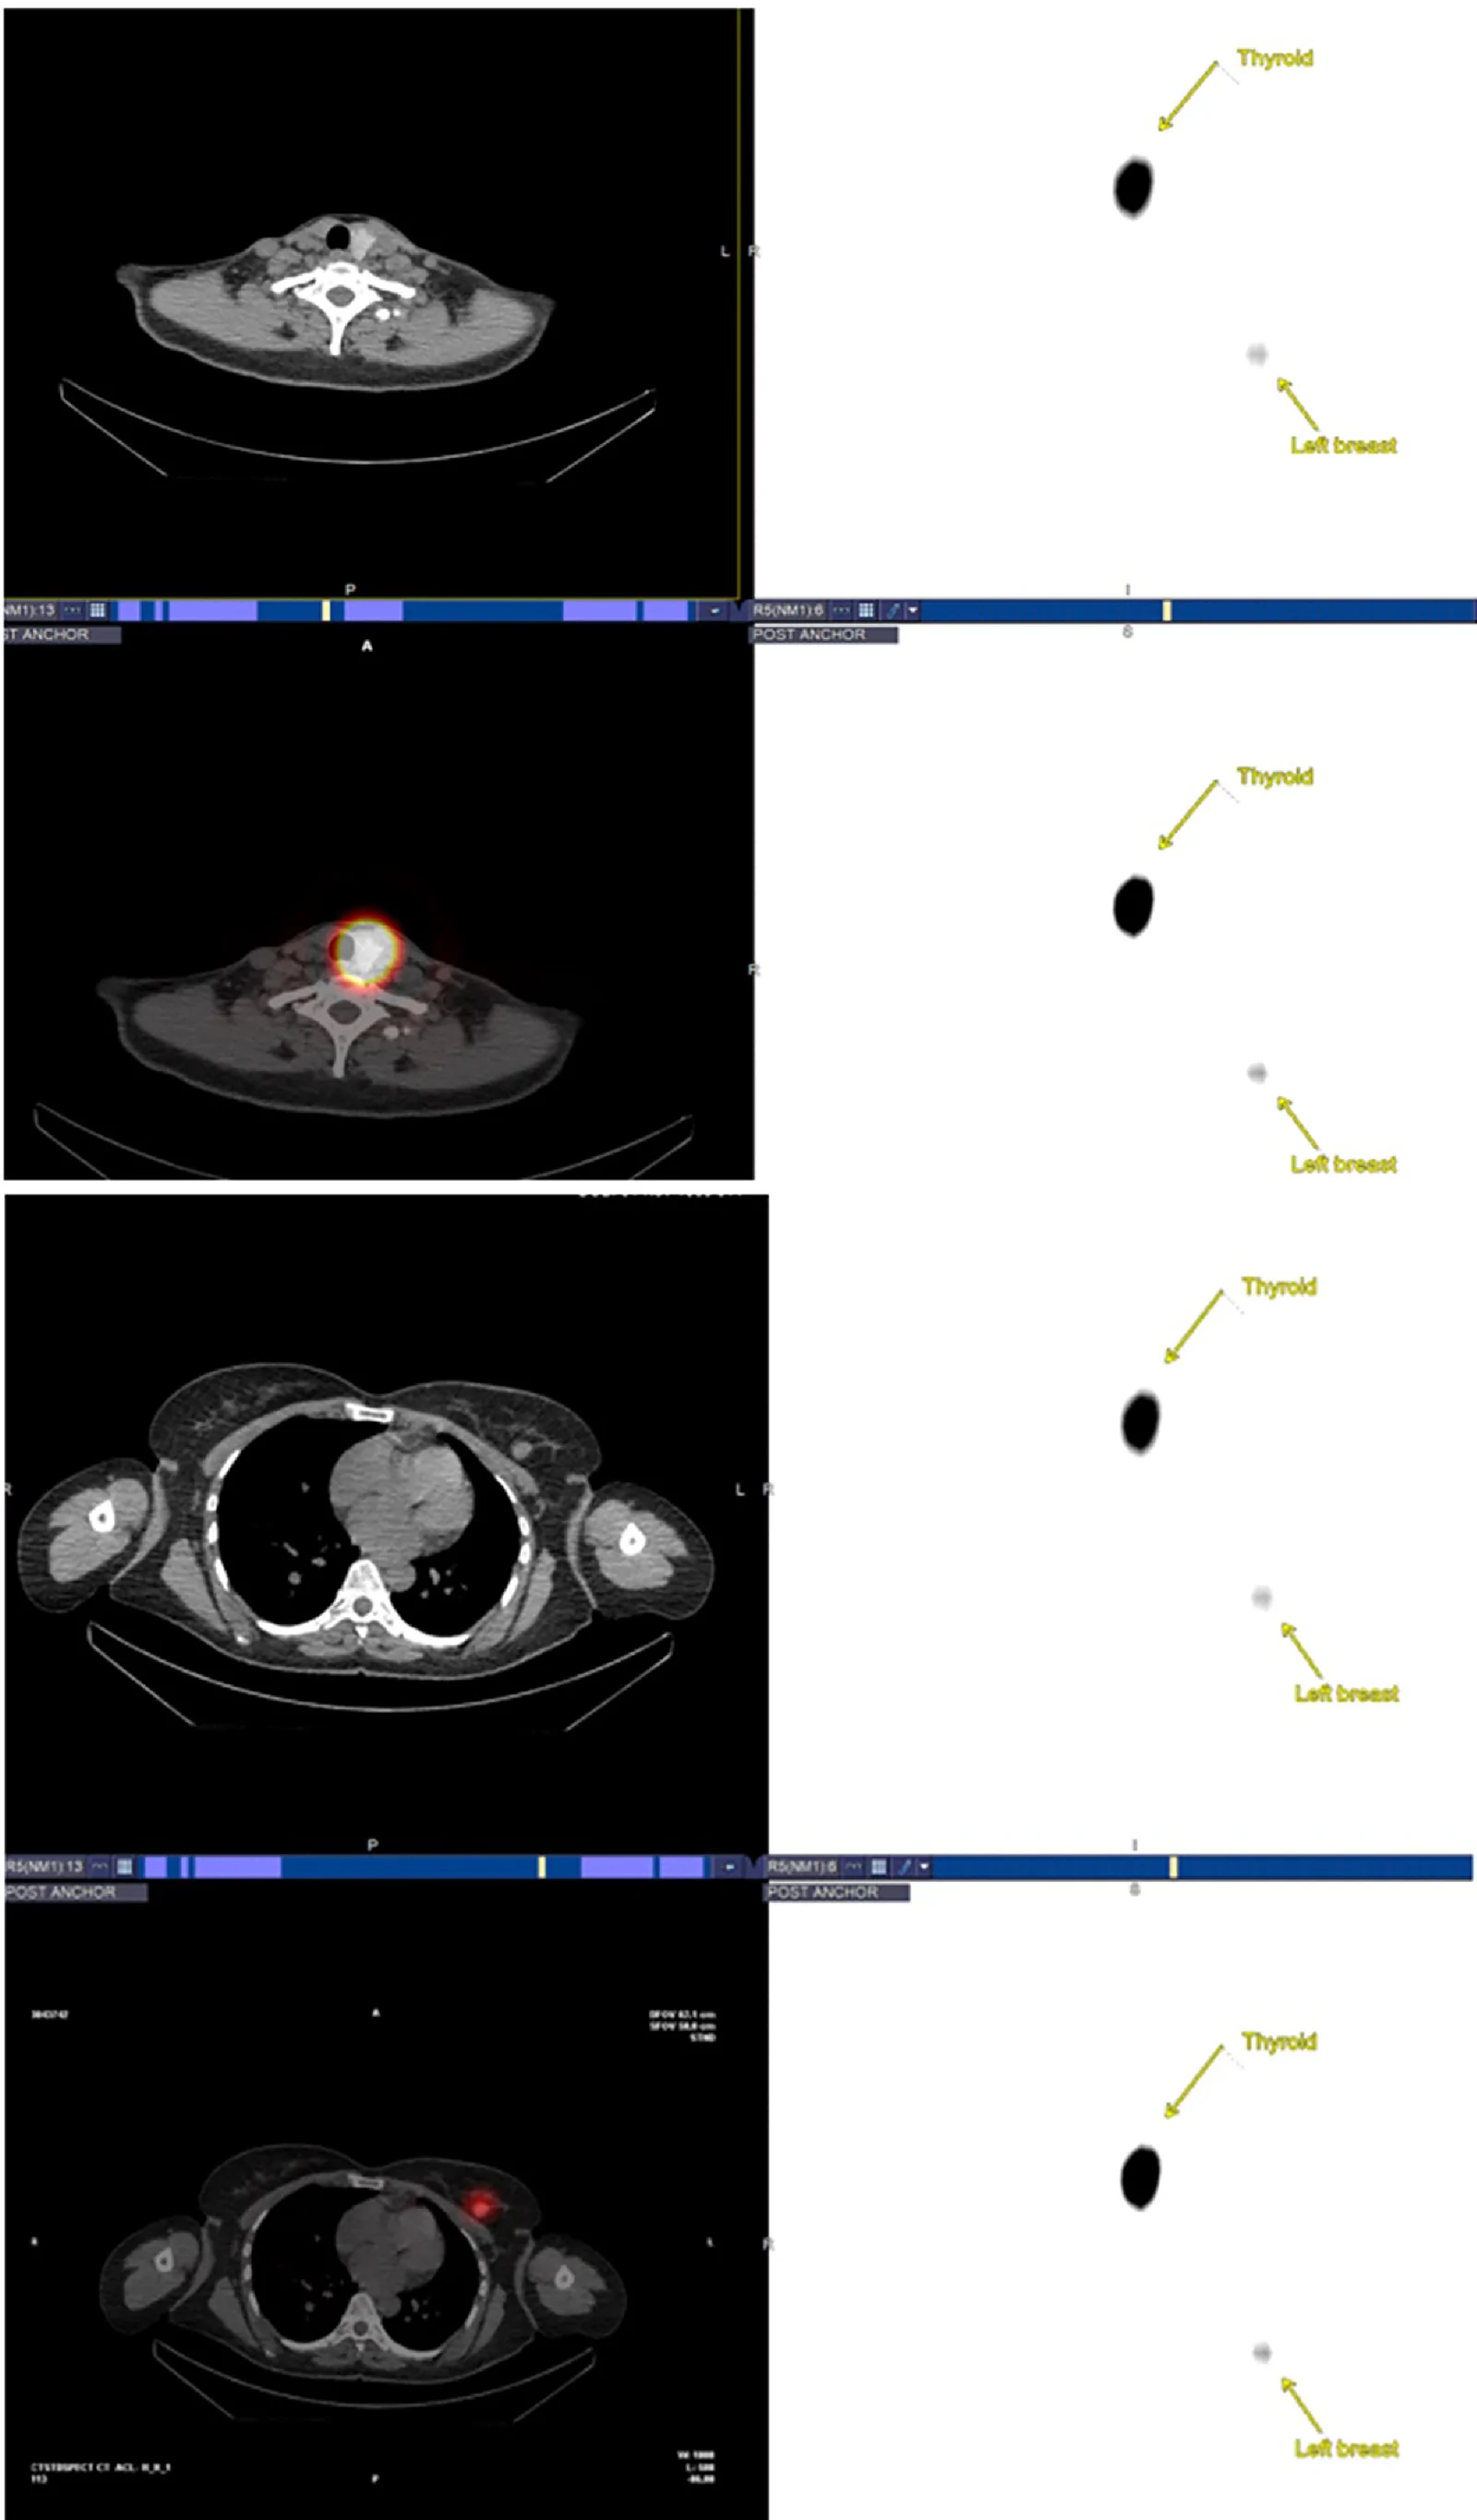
(A and B) I-123 SPECT CT identified accumulation of I-123 on the left lobe of the thyroid and within the ectopic tissue in left breast (A and B) confirming functioning thyroid tissue at both sites.
